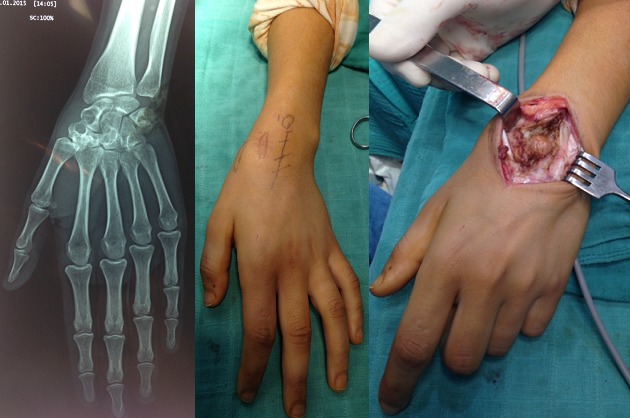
(A) radiographie du poignet de face montrant des images lacunaires au dépend du scaphoïde carpien, trapèze, trapezoide, et le grand os; (B) image clinique montrant la cicatrice de la biopsie ainsi que la voie d'abord de l'exérèse; (C) pièce opératoire
